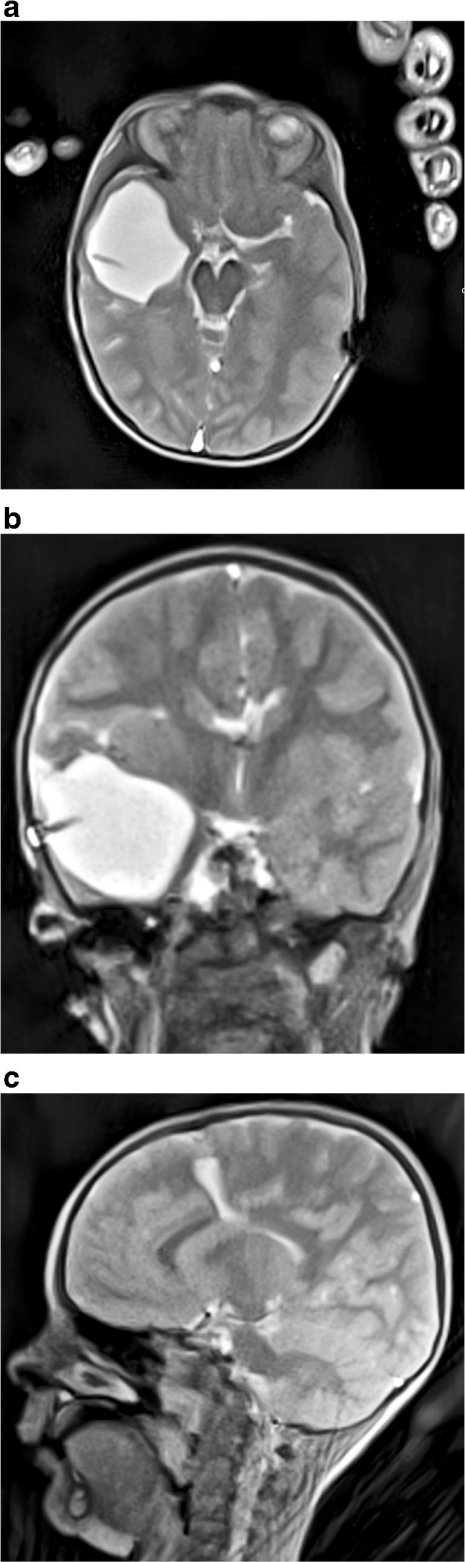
Volume coverage CT in a boy age 5 years 6 months with shunted hydrocephalus, years after relief of a right temporal abscess. a–c Volume coverage T2-W sequences are shown in axial (a), coronal (b) and sagittal (c) planes
